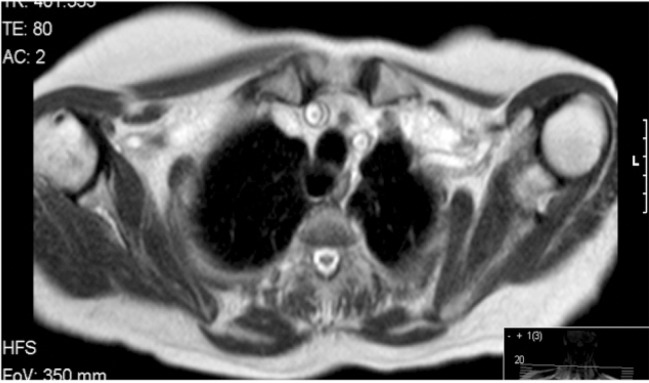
Cervical magnetic resonance imaging at 10 days after admission, showing the cystic image and the resolution of the infection.
